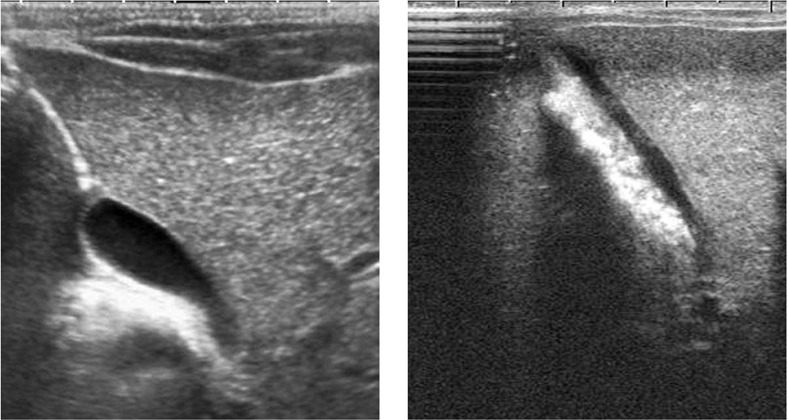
Longitudinal sonogram of the gall bladder shows hyperechoic biliary sludge filling more than half of the gall bladder with posterior acoustic shadow (a). Another sonogram, obtained after treatment, shows complete resolution of the biliary sludge (b).
